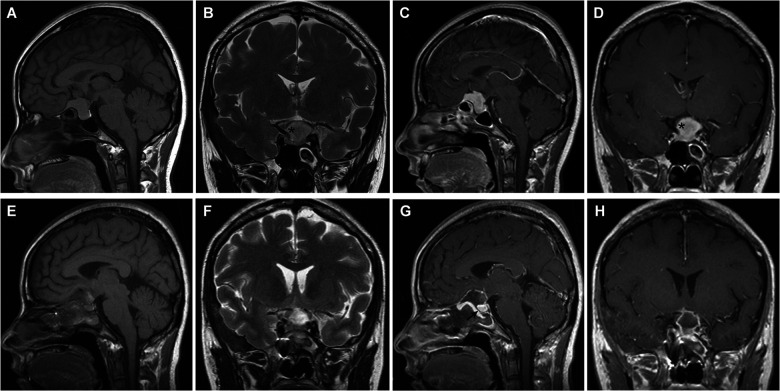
Tuberculum sellae meningioma (WHO grade I). (A–D) Preoperative MRI shows an intrasellar and suprasellar tumor with internal carotid artery encasement (>180°) (asterisk). (E–H) Postoperative MRI demonstrates gross total resection of the tumor and skull base reconstruction. The optic nerve and pituitary (arrow) were decompressed. Visual acuity and visual field were restored rapidly and pituitary function was preserved after surgery.
